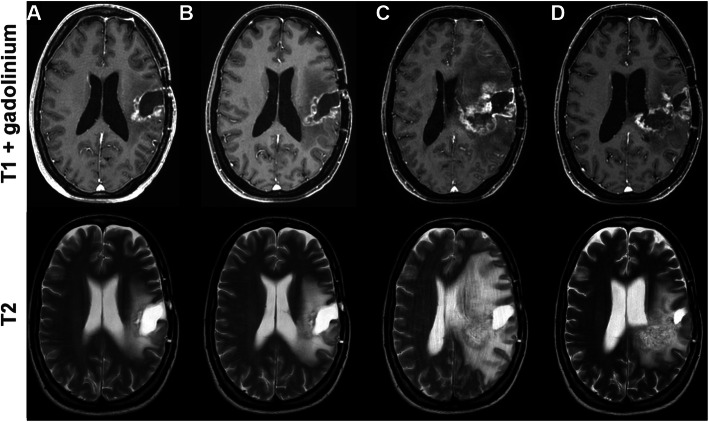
MRI scans of a 33 year old patient with IDH-wildtype glioblastoma. First (a) and second (b) MRI after resection and radiation therapy of recurrent glioblastoma showed not signs of tumor progression. Dexamethasone was started because of clinical deterioration before the third control (c) which showed a substantial increase in contrast enhancement and edema which were interpreted as late radiation necrosis. Bevacizumab 7.5 mg/kg was administered as a single-shot. d First scan 1.5 months later displayed a marked reduction of the edema while there was only a minor reduction of contrast enhancement. Diagnosis was changed from radiation necrosis to recurrent tumor
